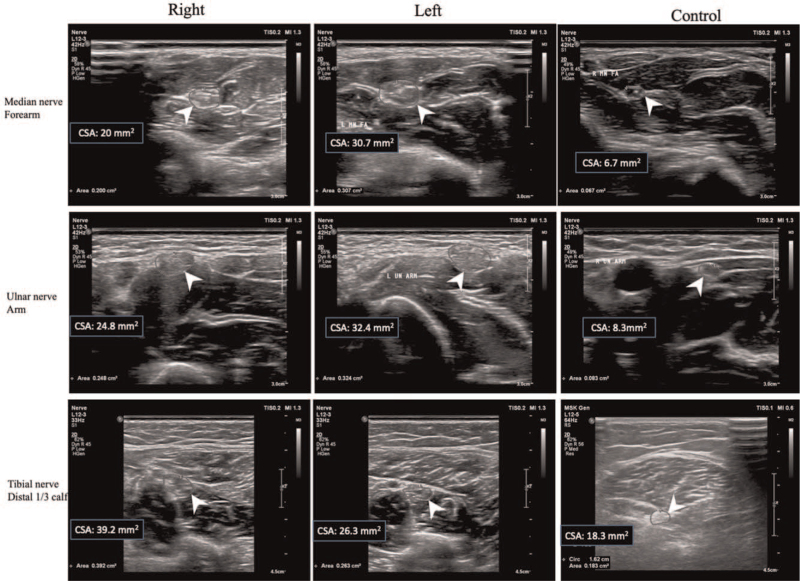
Bilateral swelling in the bilateral median, ulnar, and tibial nerves in case 2. Ultrasonography showing enlargement of the bilateral median nerves in the forearm regions, the bilateral ulnar nerve in the arm region, and the bilateral tibial nerve at the distal third leg, with an increased cross-sectional area (CSA). Swelling was not observed in the control.
